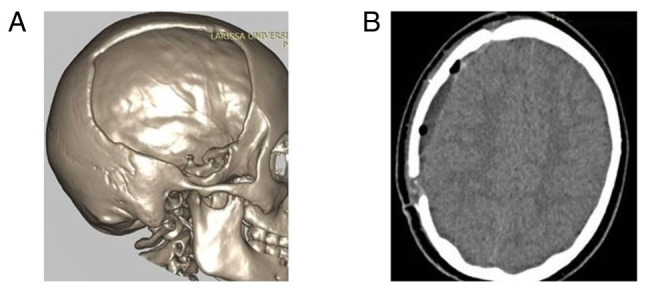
Images of the same patient as depicted in Fig. 2 are shown. (A) Post-operative 3D volume rendering following decompressive craniectomy. (B) Post-operative computed tomography scan following cranioplasty performed 9 months later with the cryopreservation of the autologous bone graft at -80˚C.
